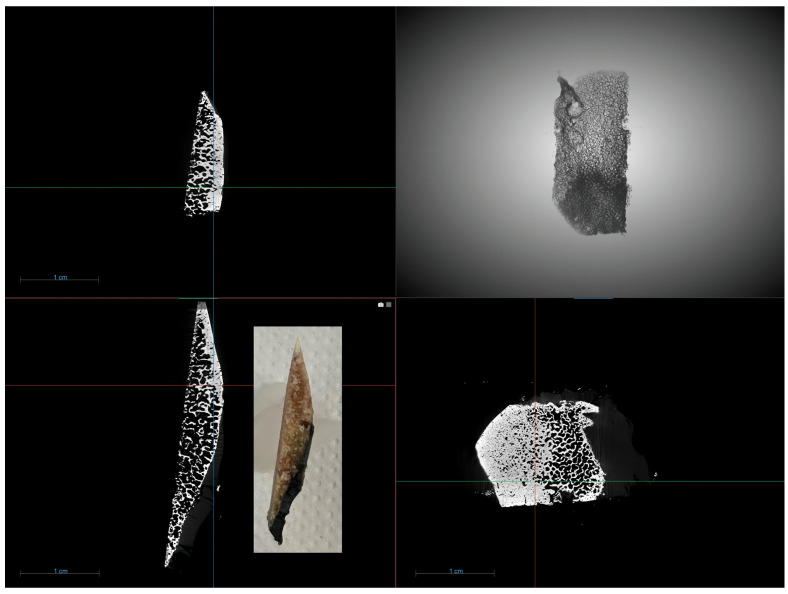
Representative nano-CT images of a selected fragment of the lateral femoral condyle from the weight-bearing region.
